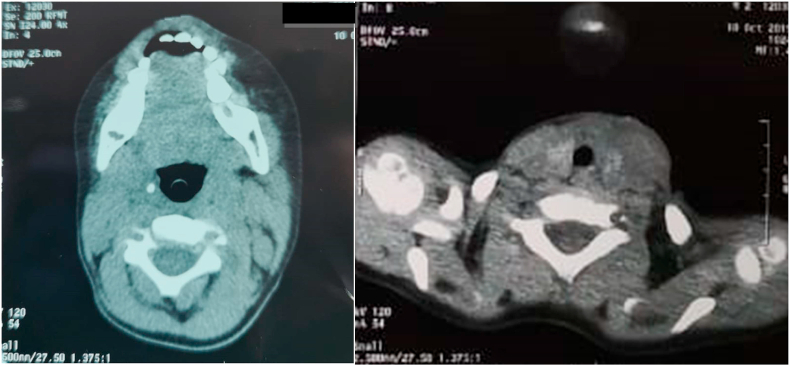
CT scan of our patient, showing the swelling of left cervical soft tissues. No gas was observed in the fistula tract nor within the thyroid.
